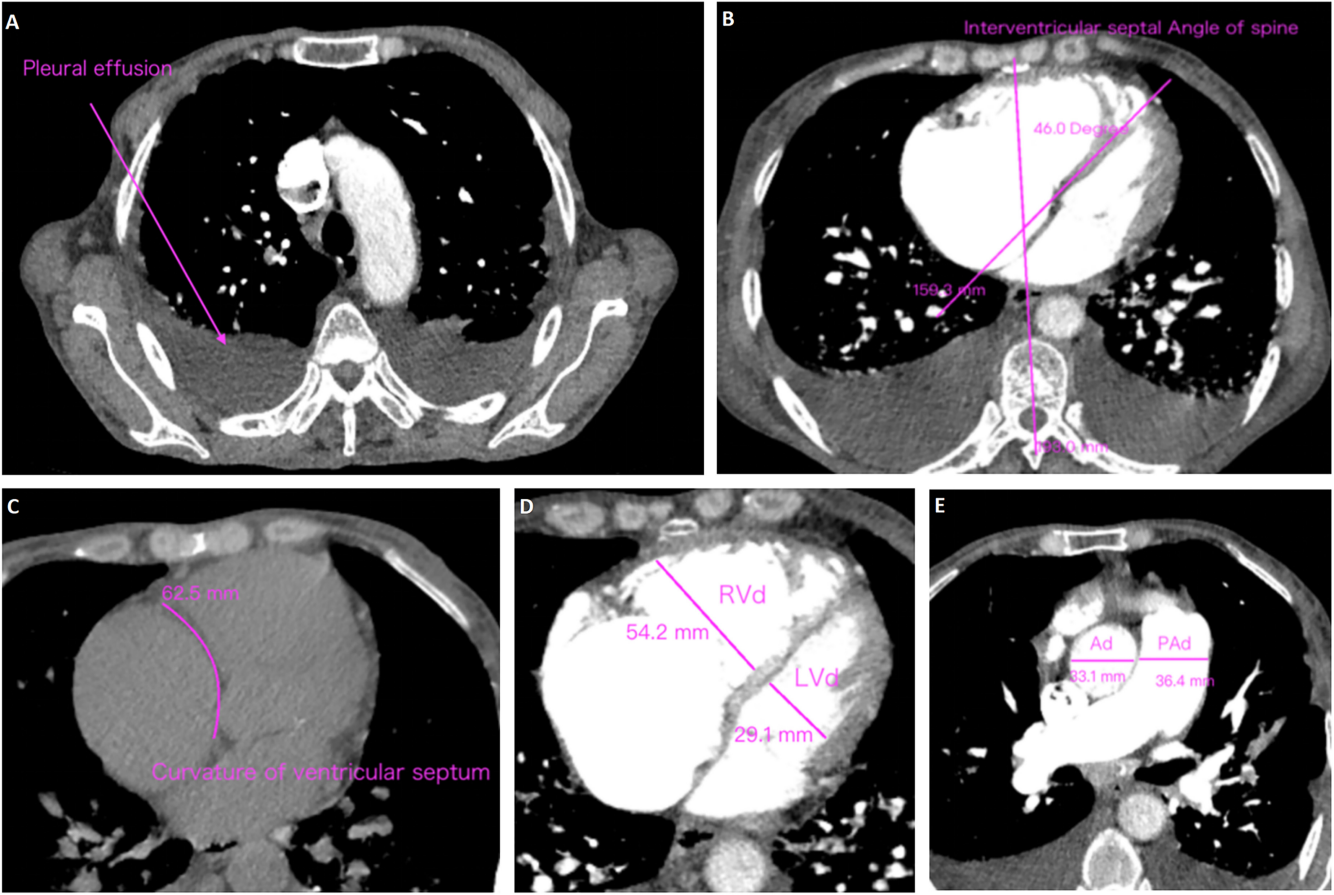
Methods of measurement and judgment of CTA signs. (A) Pleural effusion; (B) curvature of ventricular septum; (C) interventricular septal angle of spine; (D) right ventricular diameter (RVd)/left ventricular diameter (LVd); (E) pulmonary artery diameter (PAd)/aorta diameter (Ad).
